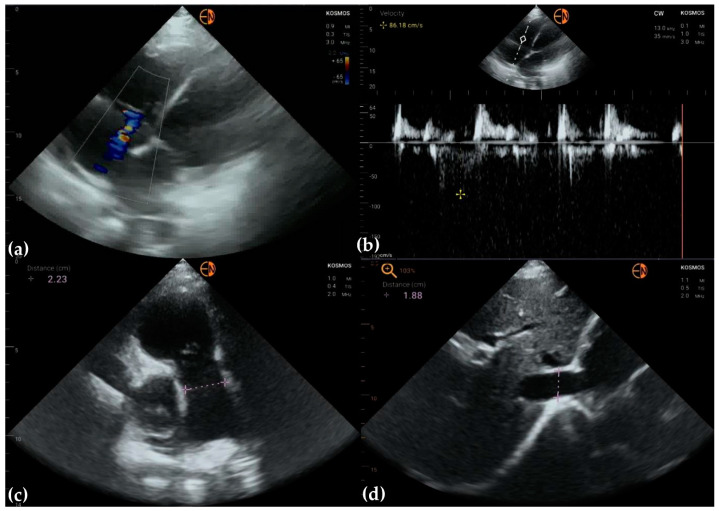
(a) Moderate tricuspid regurgitation is correctly identified on color-Doppler in a 72-year-old man presenting with decompensated heart failure; (b) continuous wave Doppler in the same patient produces faint Doppler envelope at the tricuspid valve, making pulmonary artery pressure estimation difficult; on STD, the pressure gradient between the right ventricle and right atrium was increased; (c) pulmonary artery and (d) inferior vena cava measurements.
